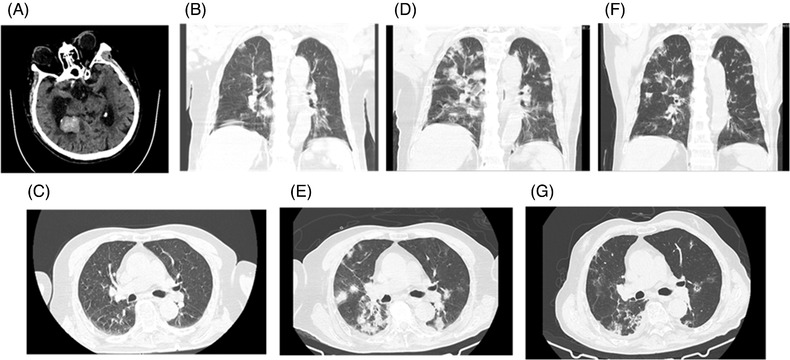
Head computed tomography and two chest CT studies in the coronavirus disease 2019 (COVID‐19) patients
